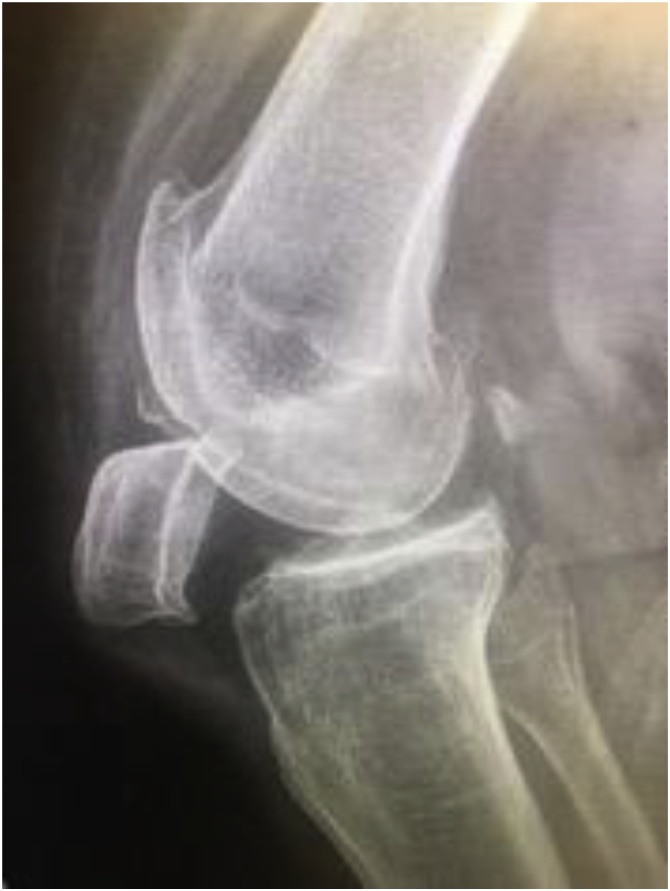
Left Knee Lateral Radiograph.
